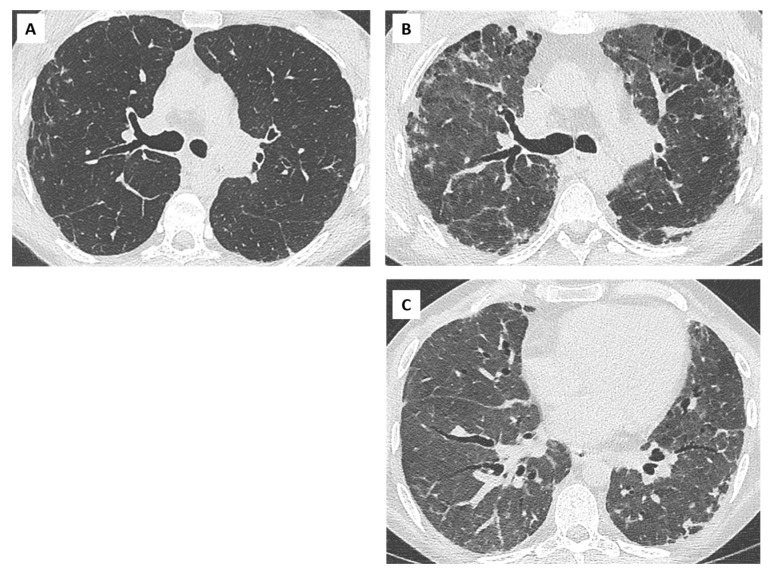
Diffuse opacities associated with RAS in a 38-year-old woman showing slowly progressive clinical symptoms and functional decline. (A) CT scan at CLAD onset, showing subtle reticular changes in the right upper lobe; (B) 2 years later, the patient has developed upper and subpleural predominant consolidation and reticulations, associated with paraseptal emphysema. Note the diffuse ground glass opacities consistent with inflammation and a possible NSIP pattern (C).
